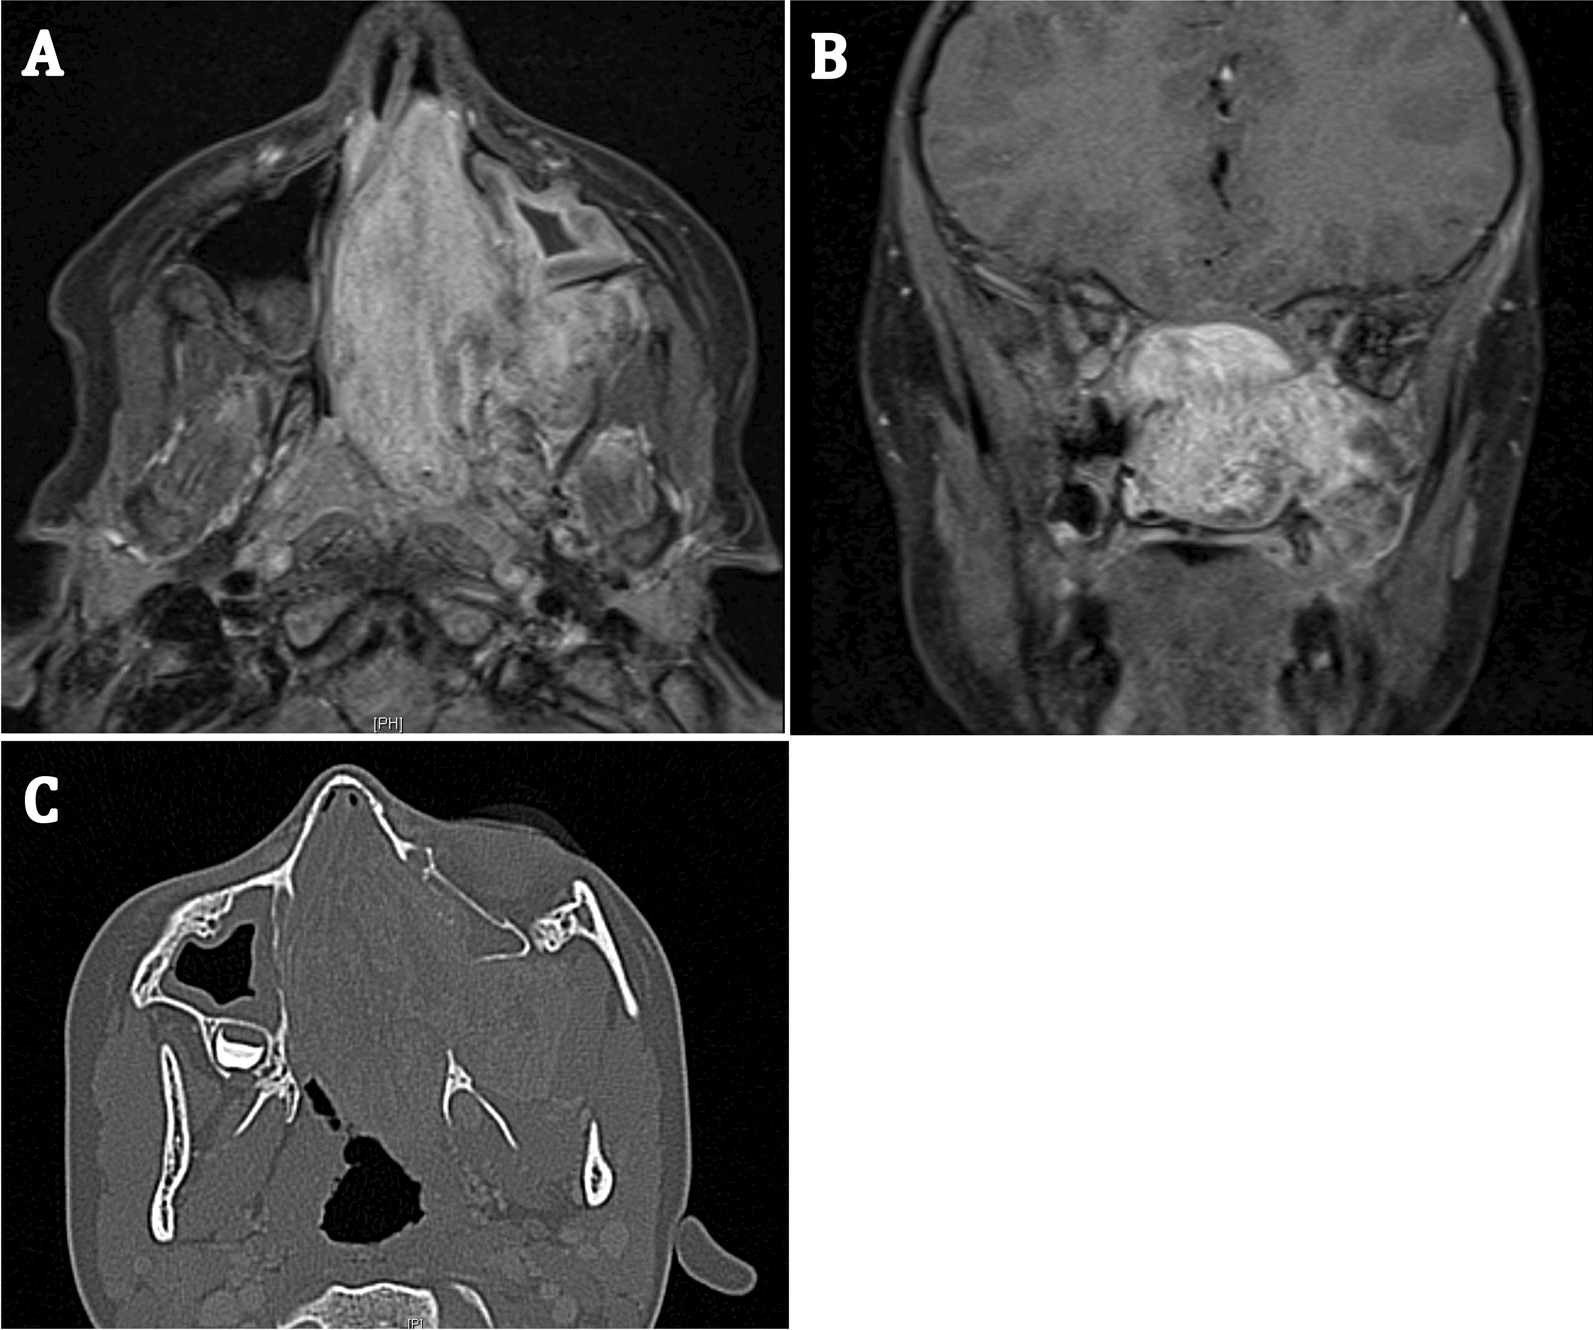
A Axial MRI view of the large JNA in the entire nasal cavity and the pterygopalatine fossa. B Coronal MRI view showing the same. C Axial CT view of the tumor
